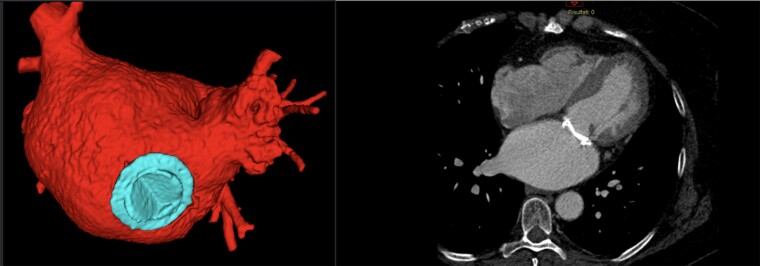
Computed tomography–based three-dimensional virtual model segmentation and computed tomography scan.
